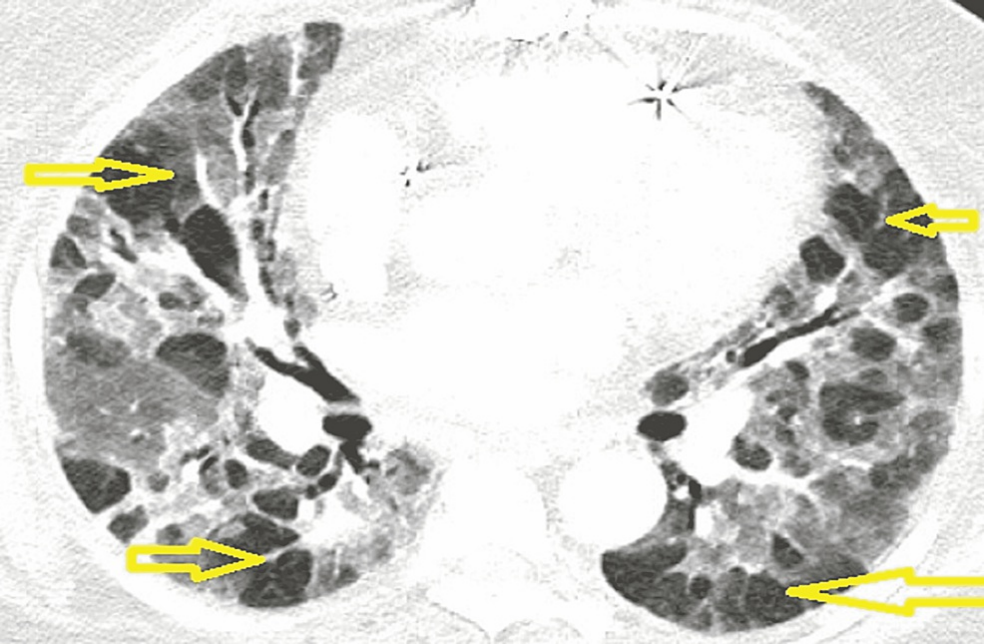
CTA PE showing severe emphysematous changes (yellow arrows) and extensive consolidation seen bilaterally. The patient was a second-hand smoker for 25 years. Her partner stopped smoking 15 years before the presentation. CTA PE - computed tomographic angiogram pulmonary embolism
